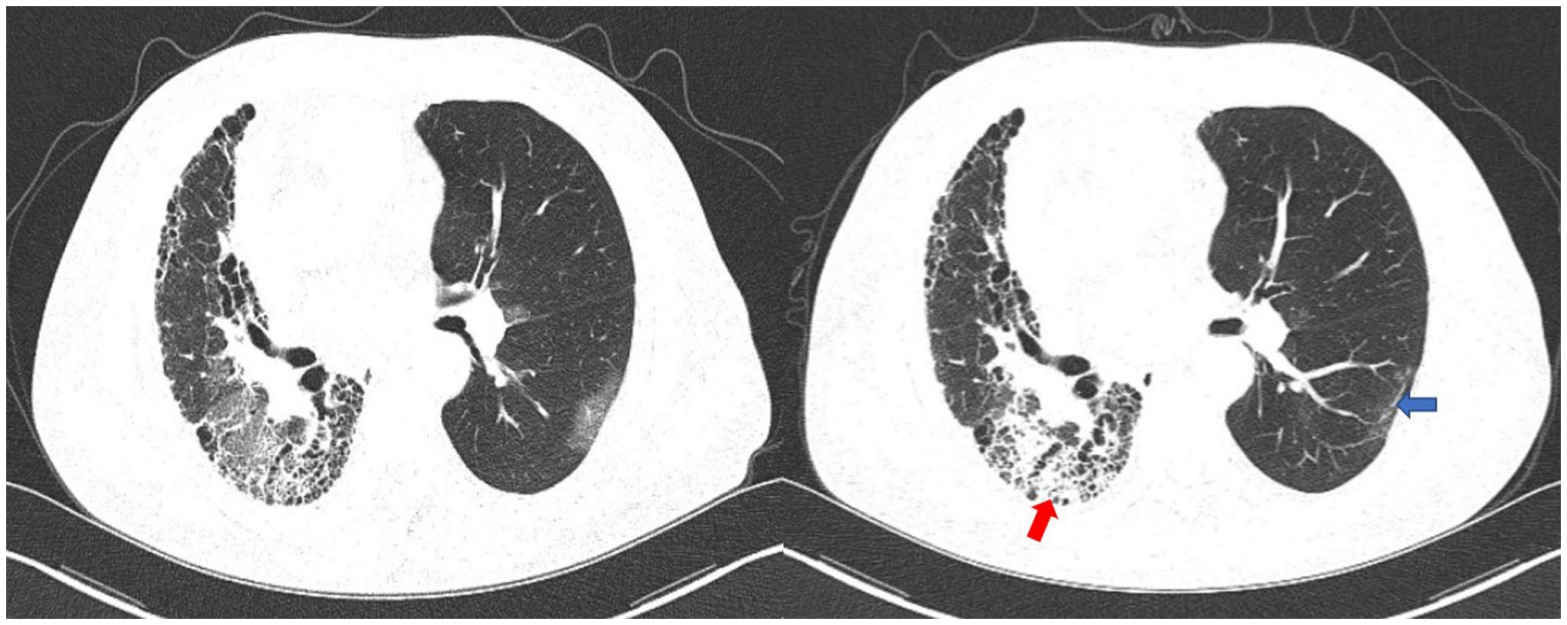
The first chest CT scan (left) and the second chest CT scan (right). The second chest CT image showed consolidation superimposed to pre-existing fibrotic changes on the lower lobe of the right lung (red arrow). The *COVID-19*-related ground-glass opacities on the left lung disappeared on the second CT, with residual tiny scars (blue arrow).
